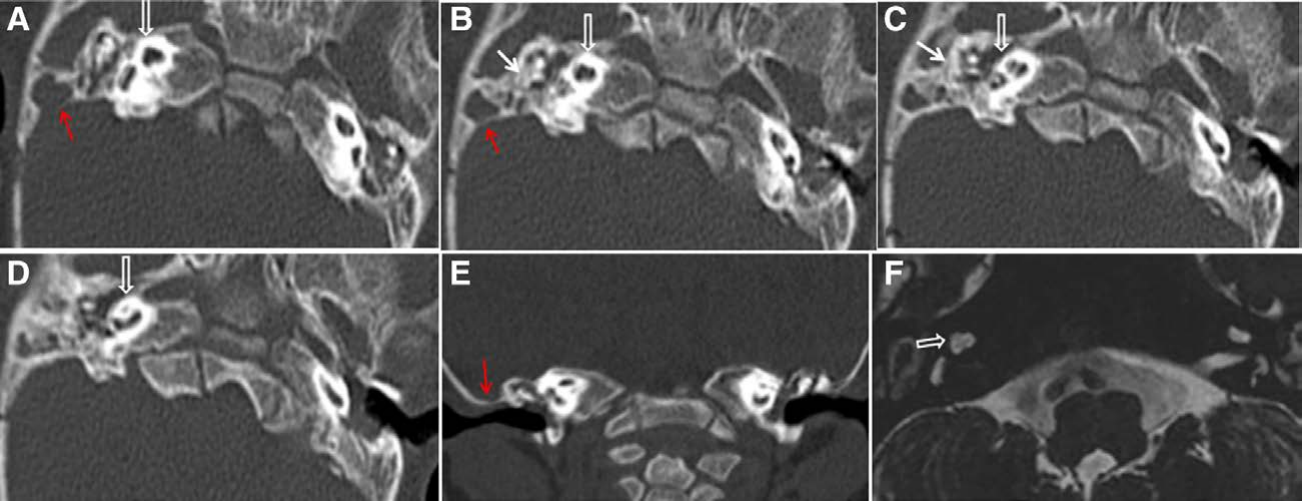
Preoperative radiologic images of the patient (CT and MRI). A-D. Axial computed tomography demonstrated bilateral low-lying tegmen (red arrows), poor pneumatization of the middle ear space (white arrow), absent lateral semicircular canals, cochlear hypoplasia (white open arrow). E. Coronal computed tomography demonstrated low-lying tegmen (red arrow). F. MRI revealed cochlear hypoplasia on the right side (white open arrow). CT= computed tomography, MRI = magnetic resonance imaging.
